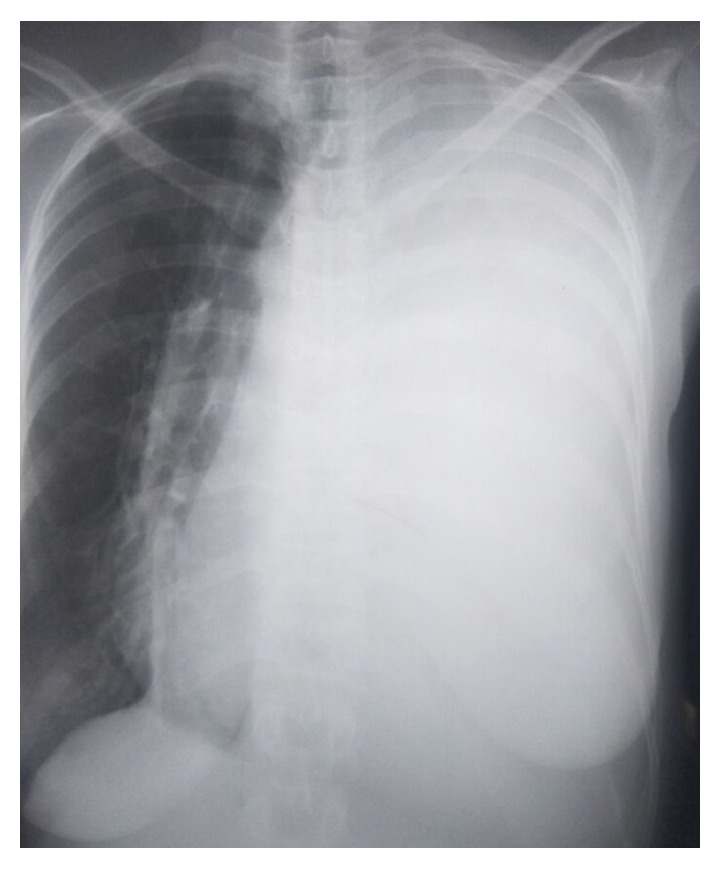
Posteroanterior chest roentgenogram showing a homogeneous opacity taking the whole hemi thoracic left field and pushing the mediastinum towards the contralateral side.
